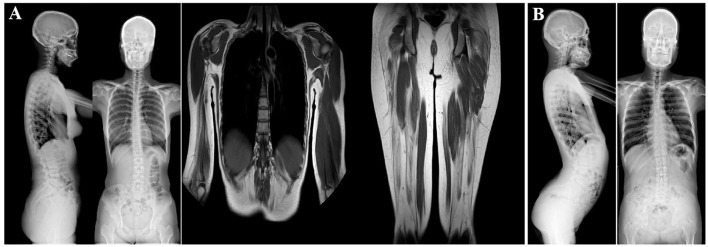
Imagological examination of two patients. (A) X-ray examination of patient 1 (III-9). She had a slightly scoliotic spine and a straightened cervical spine (left). MRI analysis of the limb muscles revealed changes in the bilateral buttocks and thighs and the right upper arm and paravertebral muscles (middle and right). These changes were consistent with the presence of muscular dystrophy. (B) X-ray examination of the spine of patient 2 (III-10). The left image shows increased physiological curvature of the lumbar spine and an upturned sacrum. The right image shows a slight curvature of the spine, a straight cervical spine.
